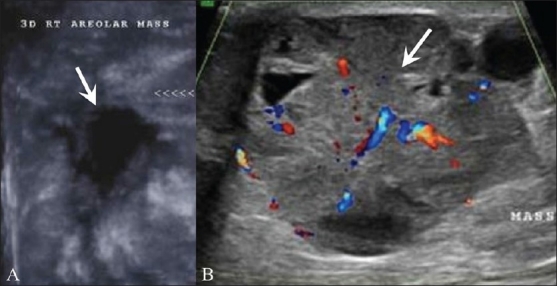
Male breast cancer. In a 80-year-old male patient with a palpable lump in the right breast, a 3D breast USG in the coronal plan (A) reveals a hypoechoic mass (arrow) with multiple spiculations, which was confirmed as an invasive ductal carcinoma on USG-guided core biopsy. In this 68-year-old male patient with a large palpable lump in the left breast, USG (B) with color Doppler shows a well-defined, vascular, heterogeneous mass (arrow) with a few cystic areas within it. USG-guided core biopsy of the mass confirmed the diagnosis of a papillary carcinoma
